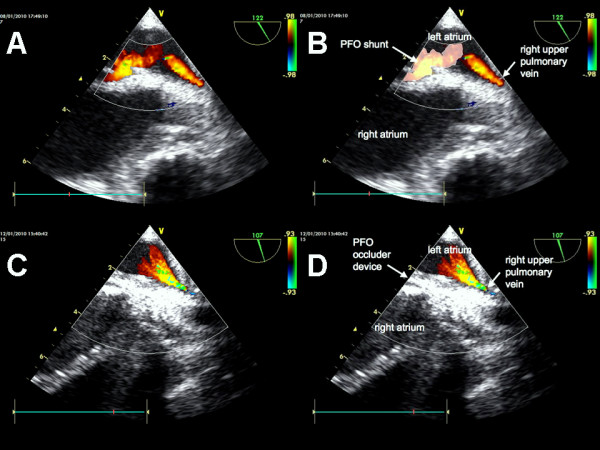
Transesophageal echocardiography: A & B before, C & D after patent foramen ovale closure with a PFO occluder device [Amplatzer PFO Occluder^®^, AGA Medical, Plymouth, USA].
